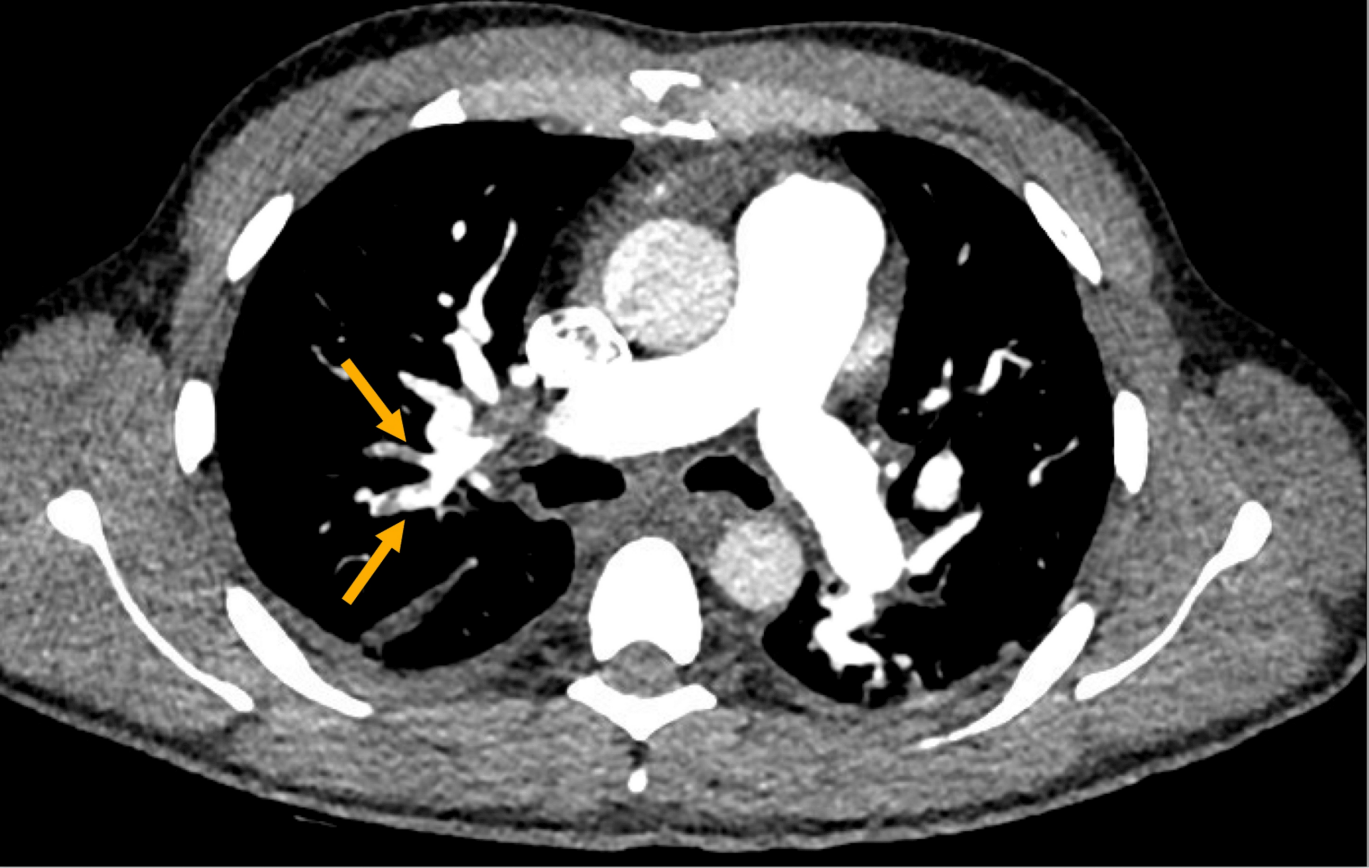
Contrast-enhanced CT pulmonary angiogram showing segmental pulmonary embolism. Axial CT image shows filling defects (arrows) in segmental branches of the right upper lobe pulmonary artery, consistent with acute embolism.
